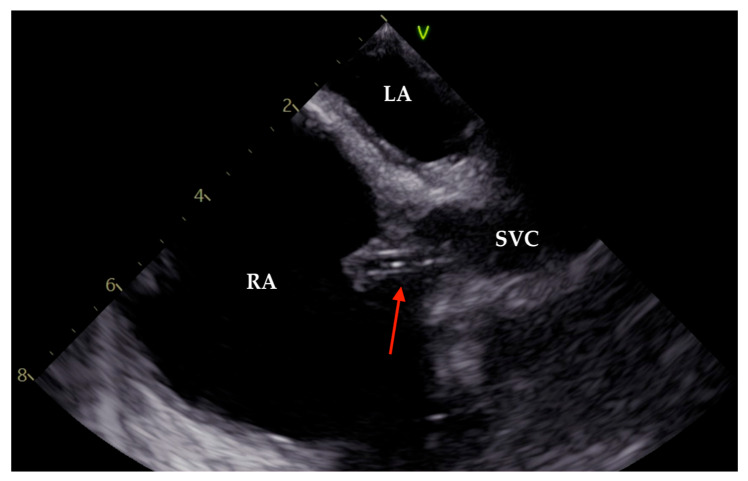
Transesophageal image (93°). Tip of the Hickmann catheter (red arrow), located in the superior vena cava and entering the right atrium, covered with the vegetation mass. LA—left atrium; SVC—superior vena cava; RA—right atrium.
